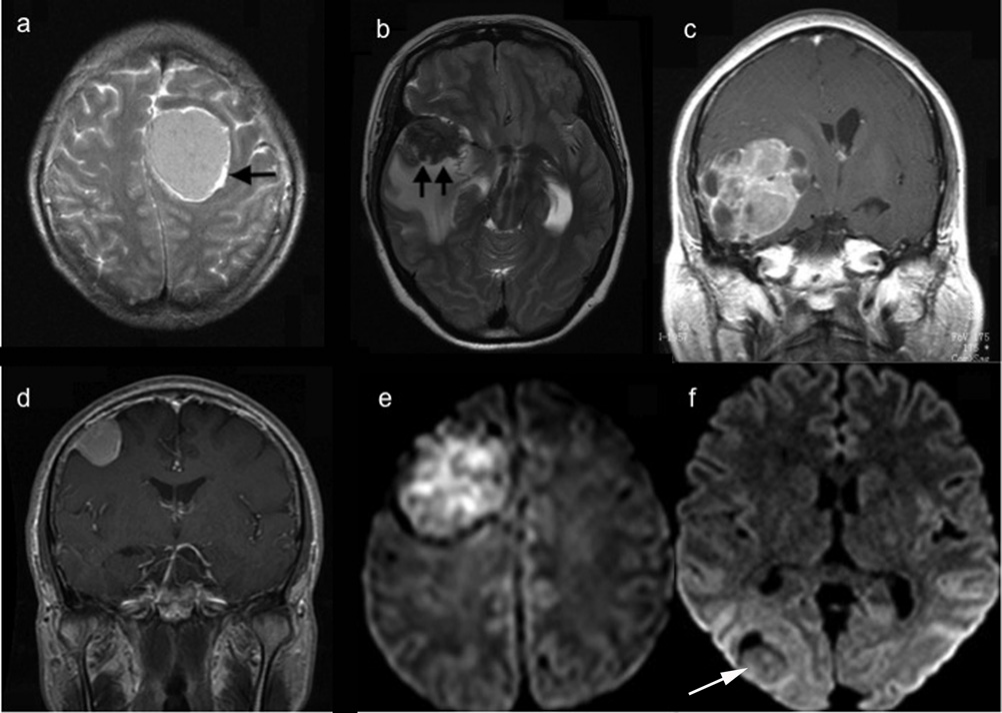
Radiologic characteristics of meningiomas in preoperative MRI. a) T2-weighted MRI demonstrating the complete arachnoid ring (black arrow) and no peritumoral edema; b) T2-weighted MRI demonstrating the disappearance of arachnoid ring (black arrow head) and peritumoral edema c) T1-weighted gadolinium enhanced MRI demonstrating heterogeneous enhancement and irregular shape of the tumor; d) T1-weighted gadolinium enhanced MRI demonstrating homogenous enhancement and regular shape of tumor; e) Diffusion weighted MRI demonstrating hyperintense lesion; f) Diffusion weighted MRI demonstrating isointense lesion.
